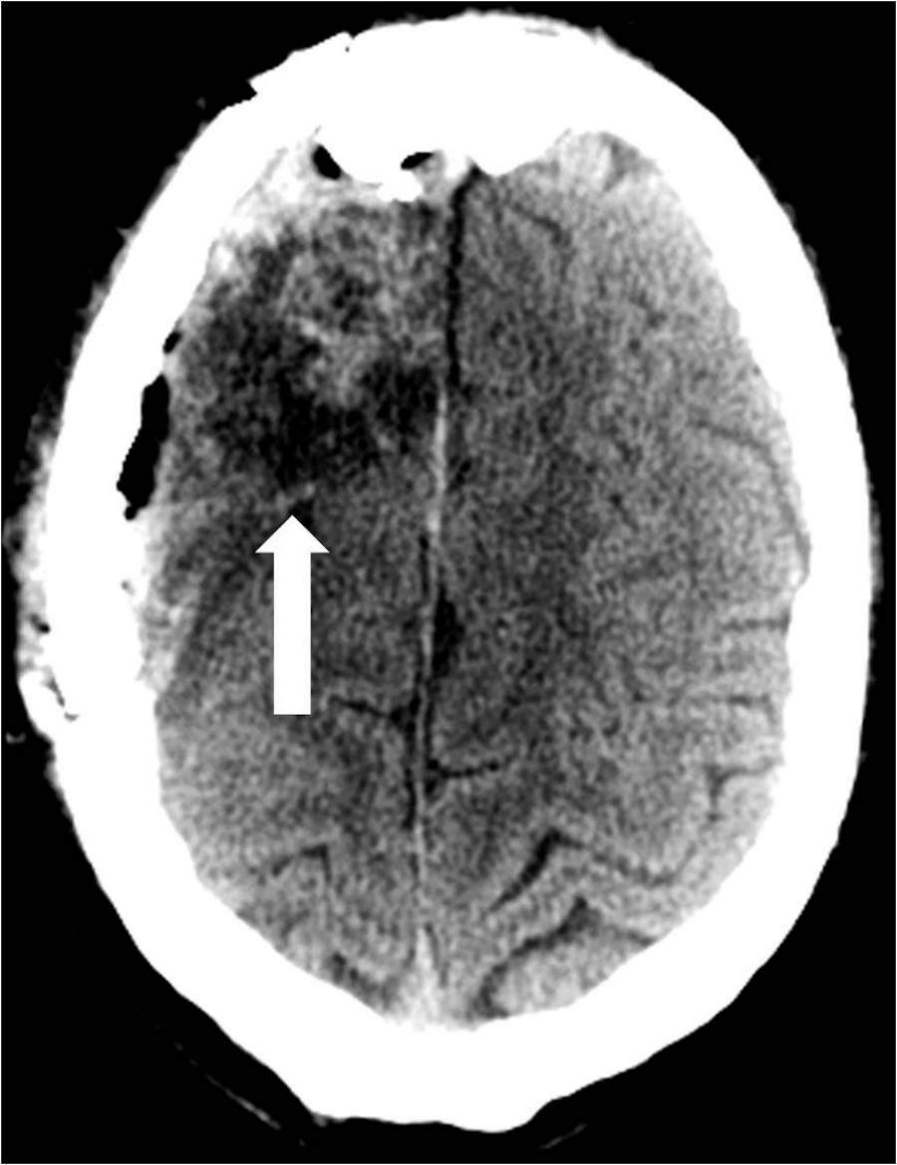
Head computed tomography on postoperative day three reveals expected changes of a right frontoparietal craniotomy with a resection cavity in the right frontal lobe (arrow).
